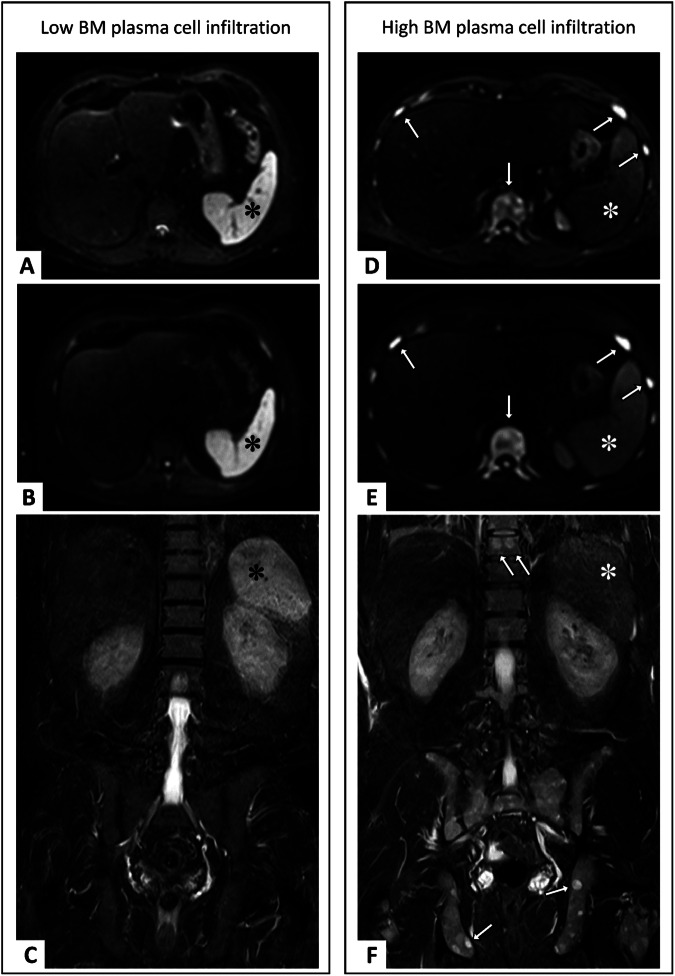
Example of two cases with different levels of bone marrow plasma cell infiltration with identical window leveling correspondingly. On the left, a physiologically hyperintense spleen (✽) is seen on axial DWI-b50 (A), -b800 (B) and coronary T2 TSE (C) in a patient with low bone marrow plasma cell infiltration (17%). On the right, there is significant loss of spleen signal (✽) on intrinsically T2-weighted b50 (D), b800 (E), as well as T2-TSE (F) images in a patient with high bone marrow plasma cell infiltration (85%). On the right, in addition, diffuse increased bone marrow signal on DWI (arrows on D and E), as well as multiple focal lesions in the pelvis and spine on coronal T2 images (arrows on F), can be noted
